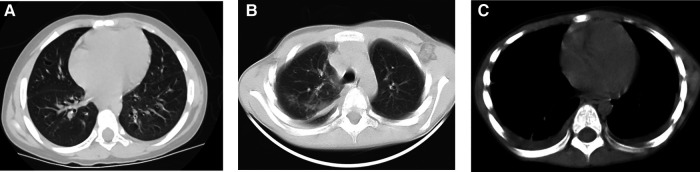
A 5-year-old boy had habit of drinking untreated water. CT of the lungs showed cystic areas (A), pulmonary exudation (B) and pleural effusion (C).
